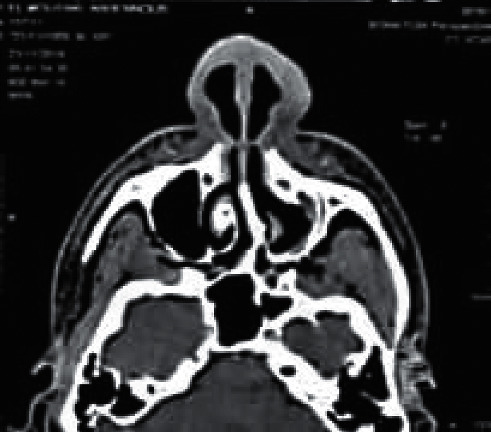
CT scan of the facial mass: no destruction of the nasal septum.
